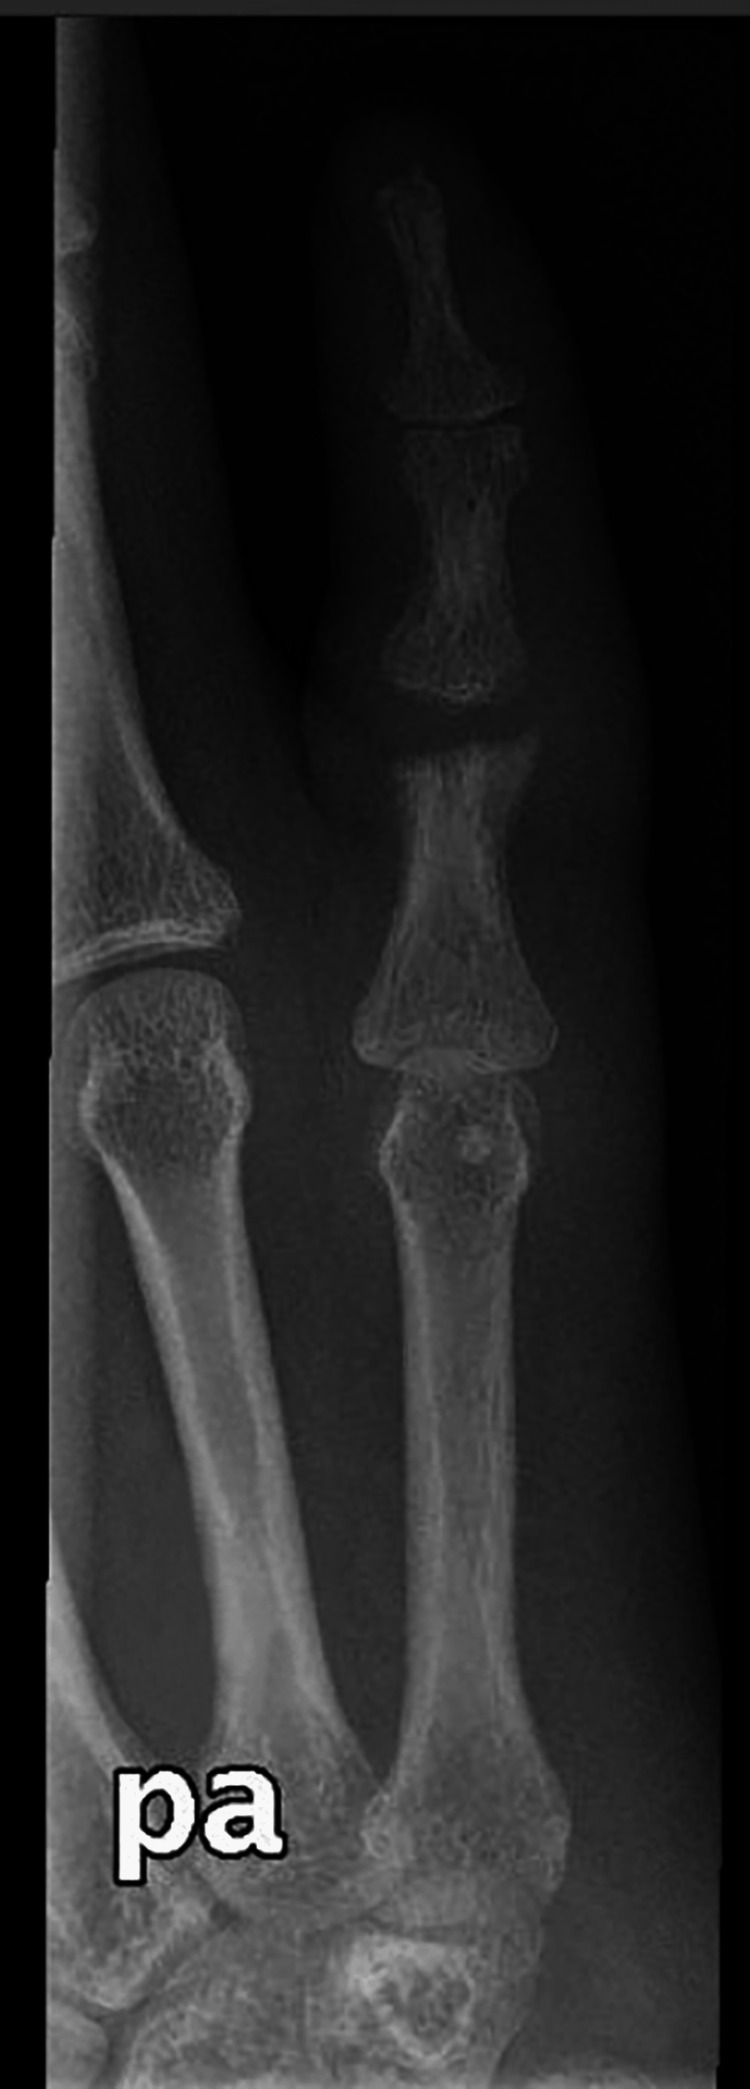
Postoperative radiographs, anteroposterior view.
